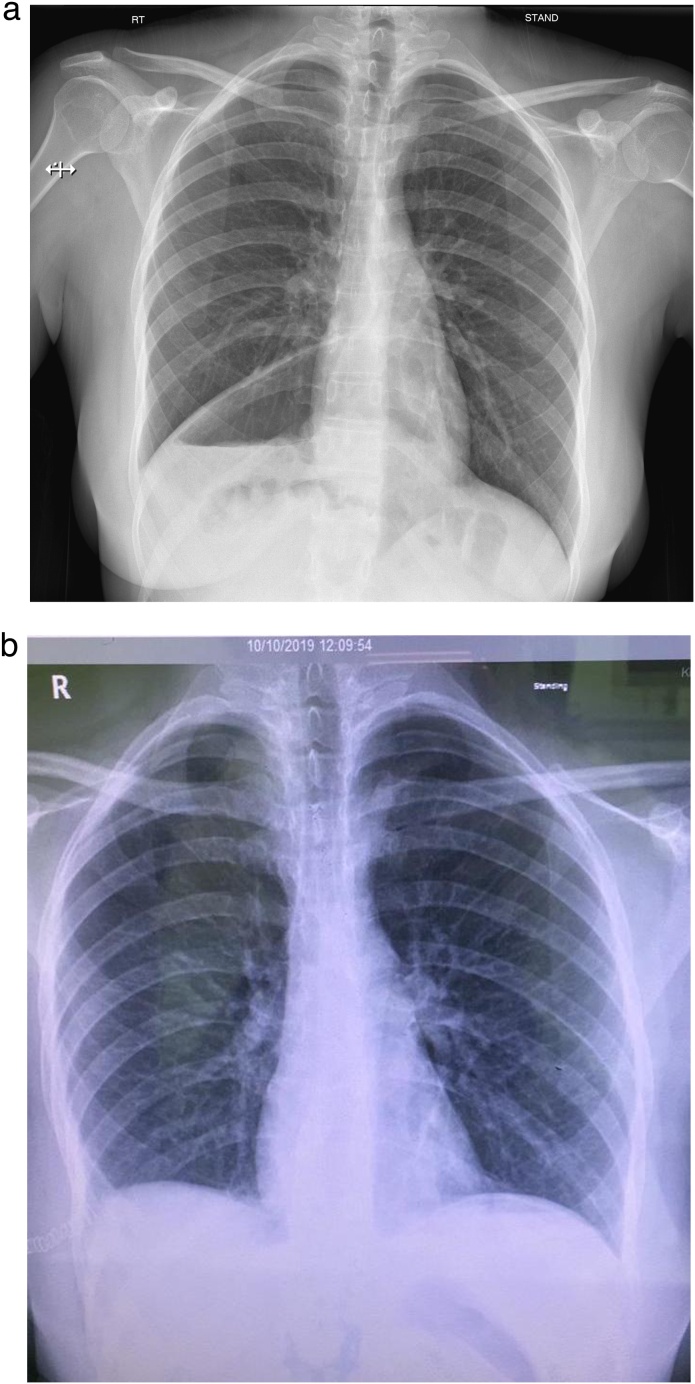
Pre-op x-ray chest with Chilaiditis Sign (a); Post-op x-ray chest (normal) (b).
